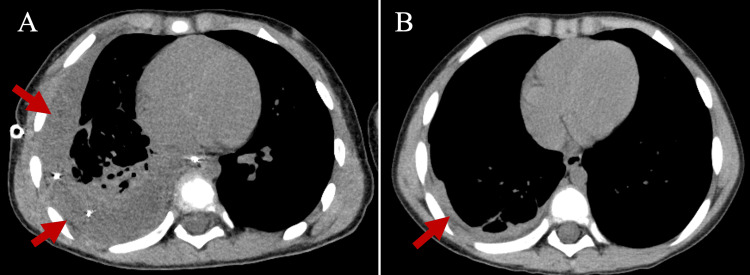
Chest computed tomography findings after treatment interventions Chest computed tomography on hospital day 6, after drainage, revealed persistent pleural effusion in the right pleural cavity and impaired re-expansion of the right lung (A). Chest computed tomography on hospital day 25, after video-assisted thoracoscopic surgery (VATS) for debridement, showed a small amount of residual right pleural effusion, with adequate re-expansion of the right lung (B).
